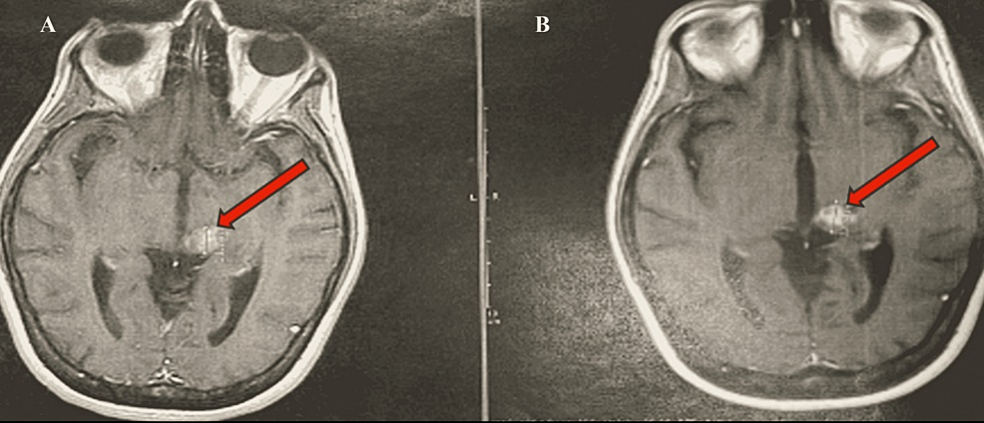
Left thalamic enhancing mass lesion with non-enhancing contrast CT measuring baseline measurement 1.28 cm × 2.06 cm × 2.84 cm (A) and cycle 2 measurement 1.6 cm × 1.6 cm × 2.56 cm (B)
